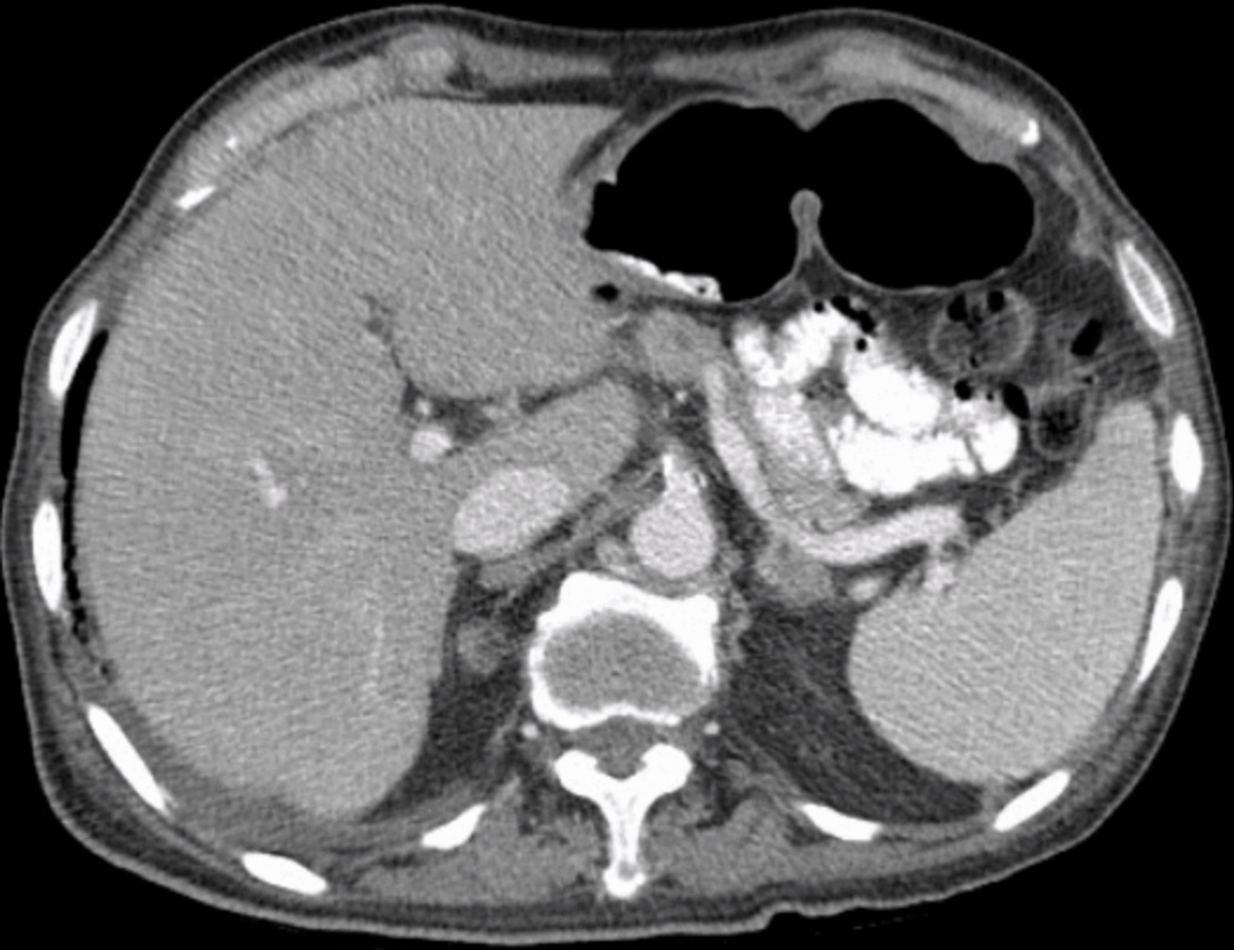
No aggressive focal hepatic lesions noted in the CT abdomen and pelvis
